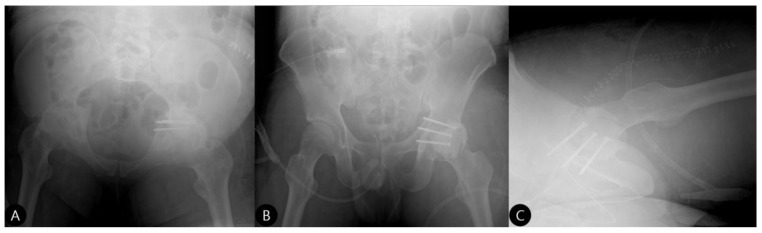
Postoperative pelvic radiographs. (A) Outlet, (B) inlet, and (C) cross-table lateral views demonstrate successful reduction in the hip joint and the position of the cement-augmented construct.
